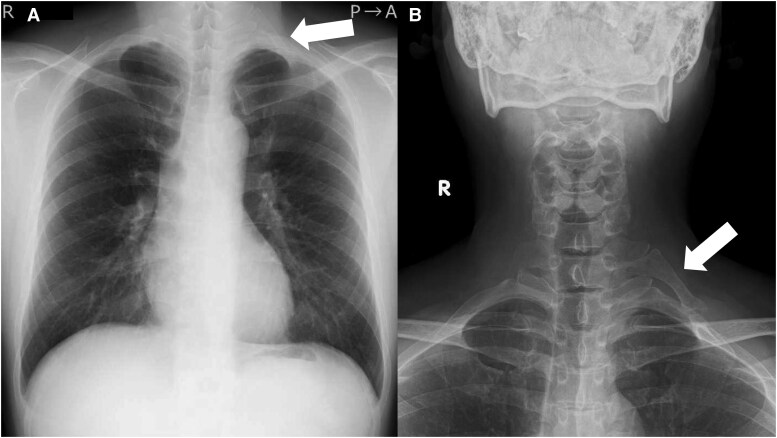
Chest (*A*) and cervical spine (*B*) radiography revealed a cervical rib on the left side (white arrows).
